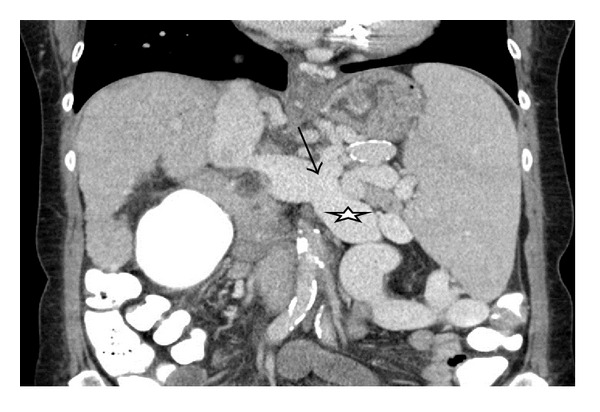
Preoperative CT imaging showing the splenorenal shunt (arrow) and a splenomegaly (left renal vein = star).
